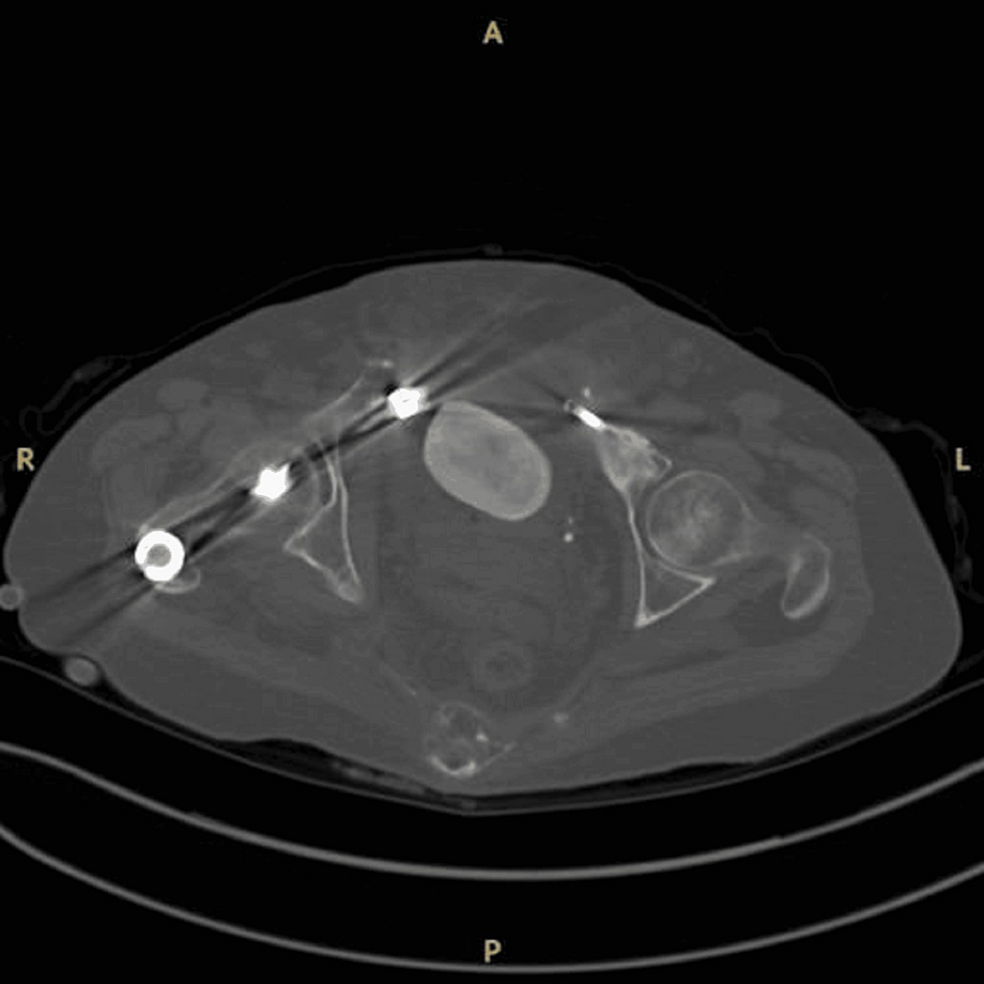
Abdominal computed tomography showing a 5-6 cm bladder stone.
